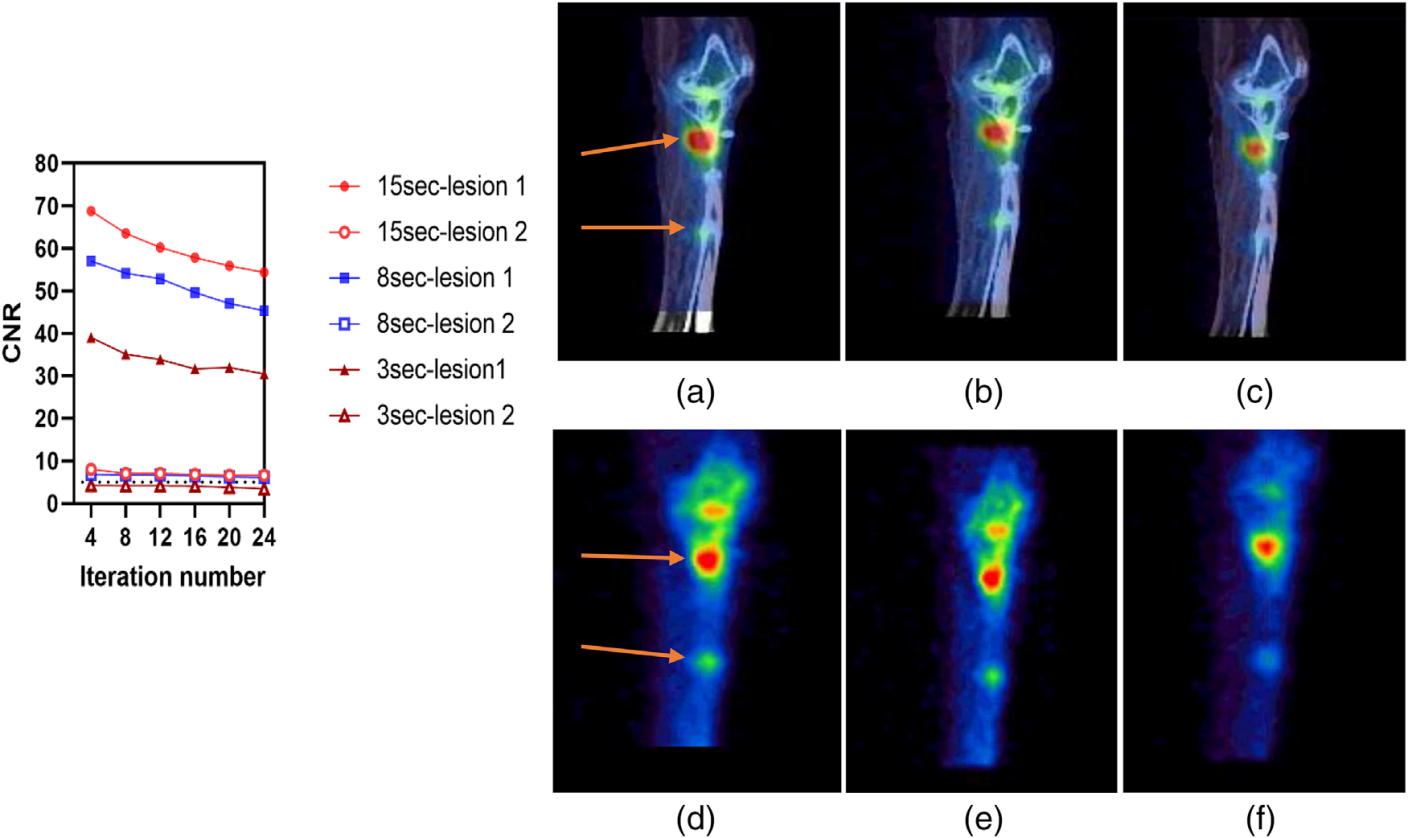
Left: contrast‐to‐noise ratio (CNR) for lesion 1 and lesion 2 for the first clinical case at different acquisition time. Right: single‐photon emission computed tomography/computed tomography (SPECT/CT) and sagittal SPECT images at: (a and d) 15 s, (b and e) 8 s, and (c and f) 3 s. The dotted line on the graph represents CNR = 5 (Rose criteria). All reconstructions used 12 iterations, eight subsets, and an 8 mm Gaussian filter
